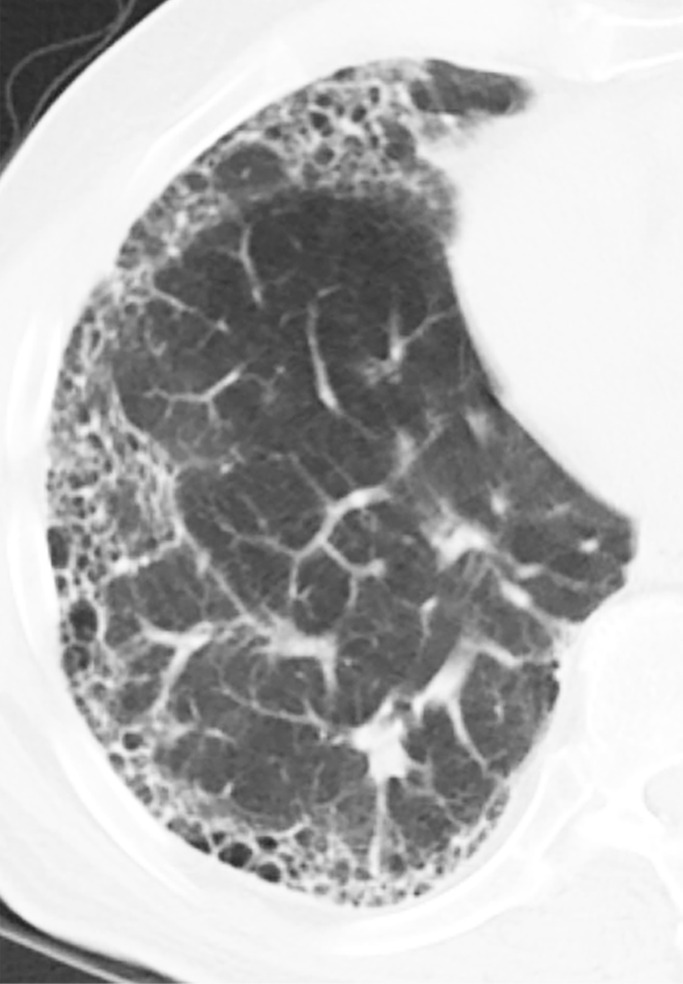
Honeycomb cysts and paraseptal emphysema. (a) Zoomed view of axial CT demonstrates classic honeycomb cyst formation with stacking subpleural cysts and associated adjacent fibrosis in a patient with idiopathic pulmonary fibrosis. (b) Zoomed view of axial CT in a different patient demonstrates paraseptal emphysema, characterized by a single layer of subpleural cysts, without any evidence of associated fibrosis in a patient with a history of marijuana cigarette smoking. The cysts of paraseptal emphysema are typically more than 1.0 cm in diameter, larger than honeycomb cysts, and have thinner walls.
